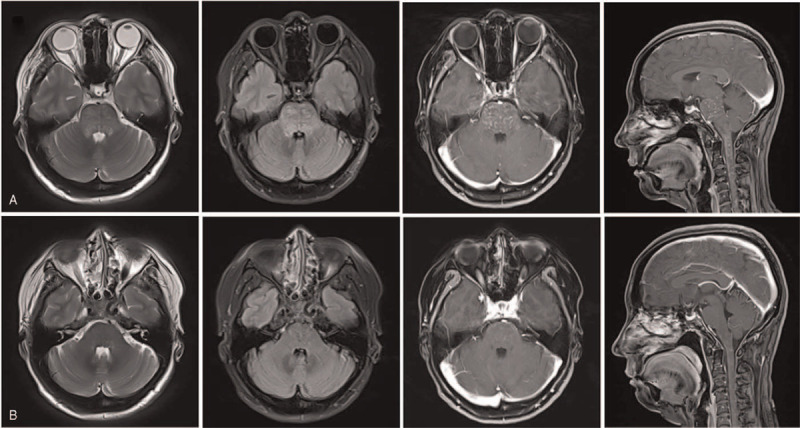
Representative T2, FLAIR, and T1 post-gadolinium MRI images of the patient. (A) T2, FLAIR, and T1 post-gadolinium MRI images of the patient before treatment. (B) T2, FLAIR, and T1 post-gadolinium MRI images of the patient after treatment. MRI = magnetic resonance imaging.
